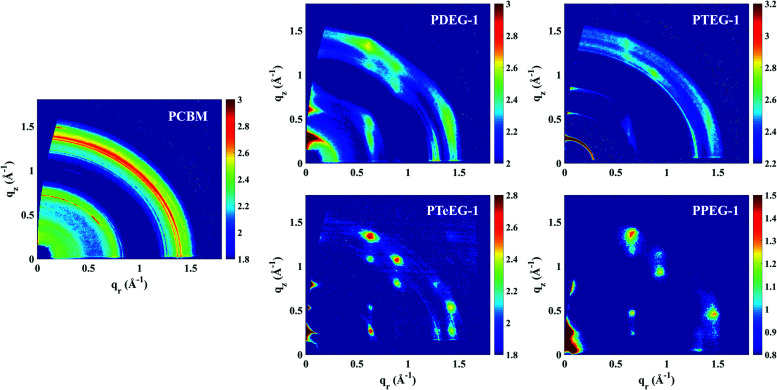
GIWAXS patterns for the C_60_–EG derivatives spin coated from chloroform solutions. The GIWAXS for a PCBM thin film is also reported for comparison. Note that the PPEG-1 sample was measured using the lab X-ray instrument, while all the others were measured at the synchrotron.
